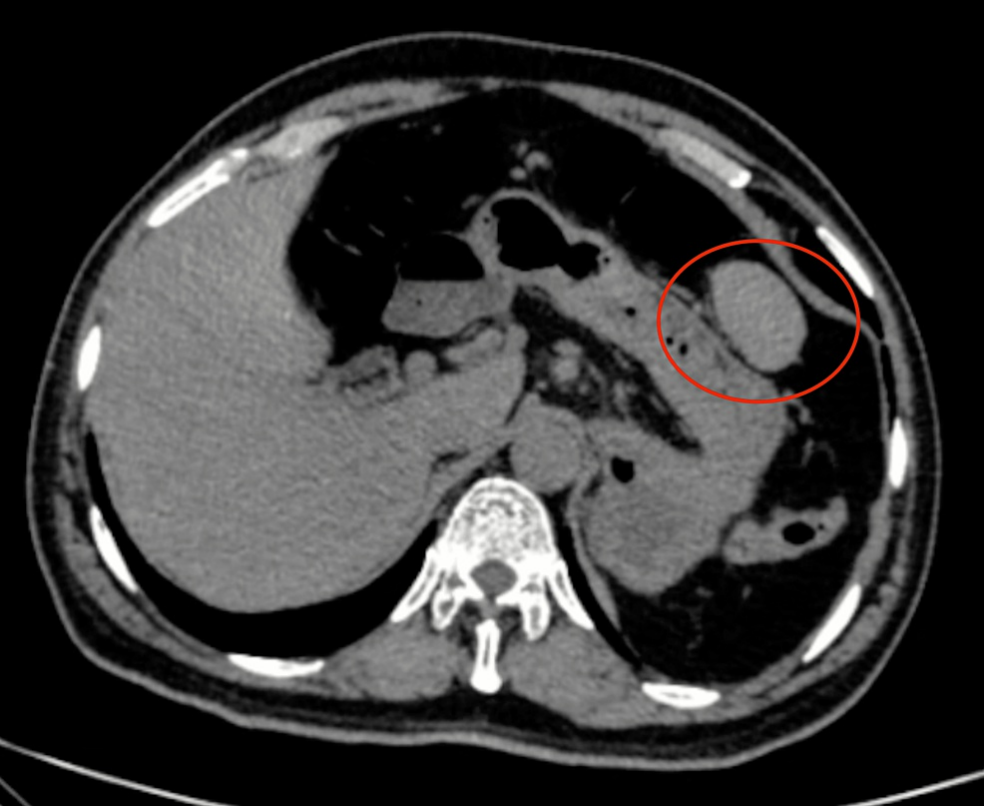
Lower cut of the same CT showing the lesion
